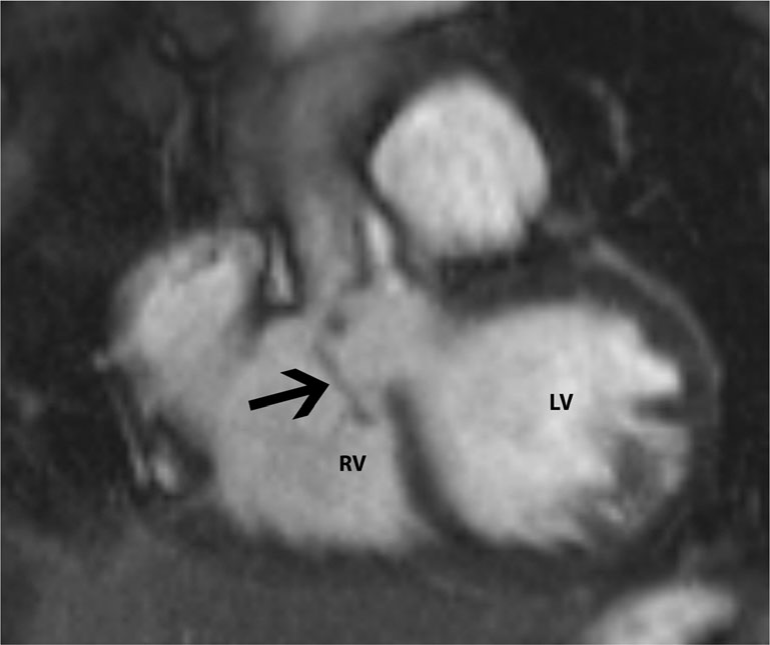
Aneurysm of the membranous septum. Coronal steady-state free precession (SSFP) MRI image shows an aneurysm of the membranous septum, formed by adhesion of the septal tricuspid leaflet to a rim of membranous ventricular septal defect (VSD). This results in partial or complete closure of the VSD with or without an aneurysm formation
